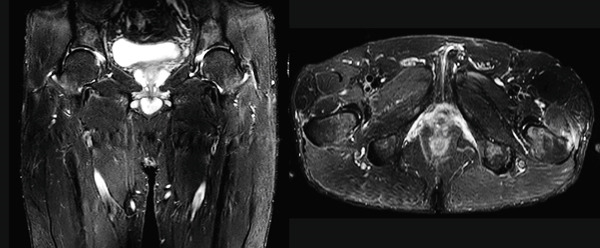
Case 1. One year after surgery, coronal and axial MRI with STIR images of bilateral hips and femurs.
